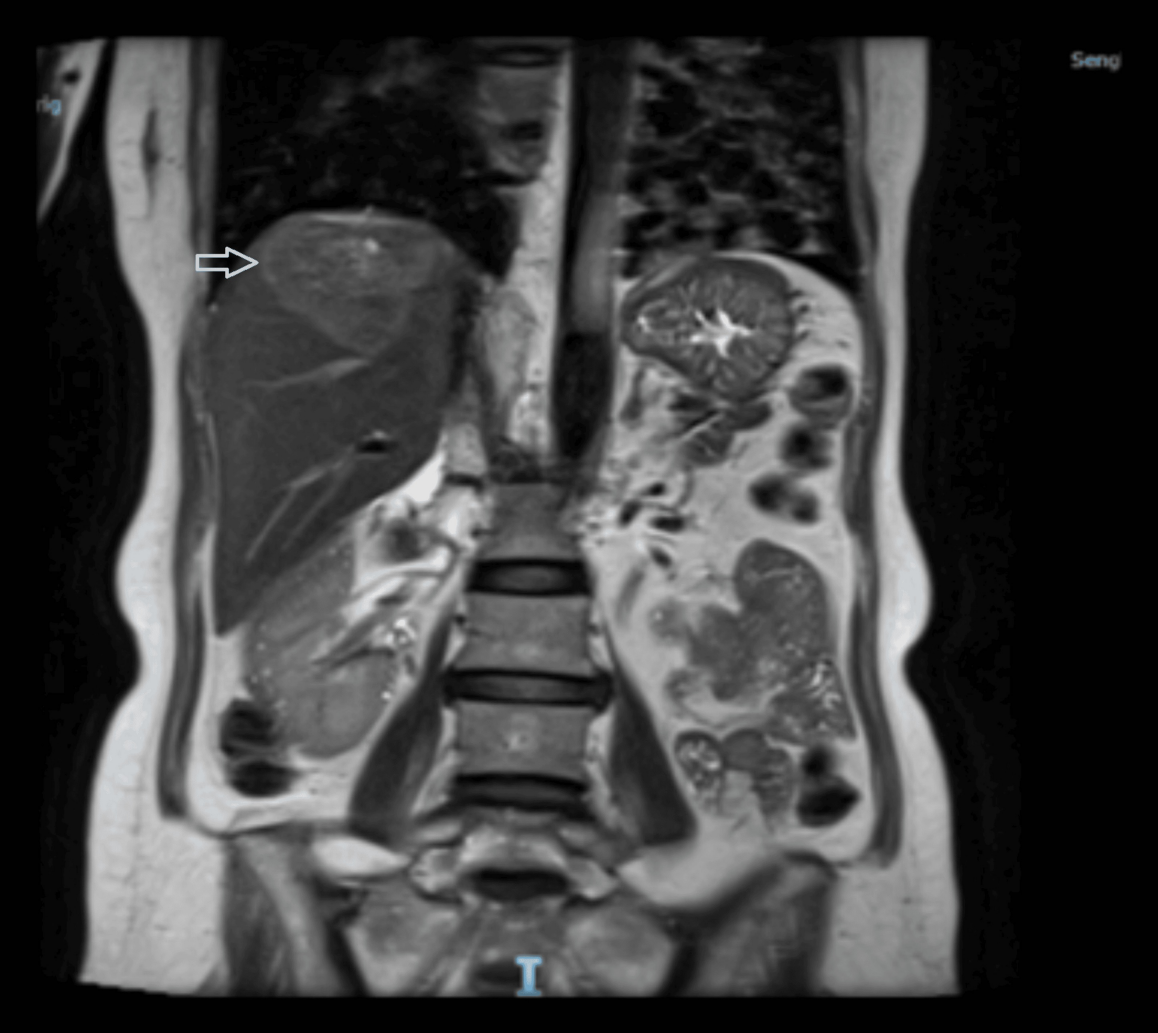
Coronal view of MRI liver showing the liver mass. Note it is located quite close to the diaphragm
